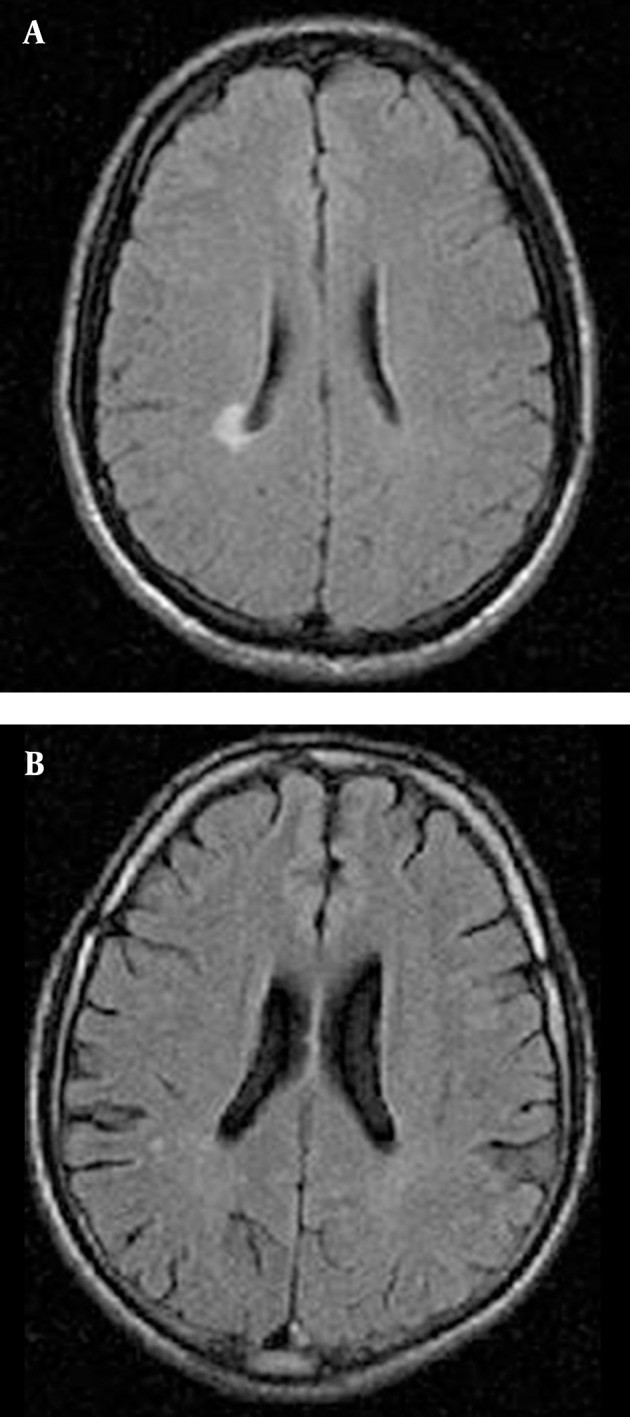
Axial FLAIR images. A) MA dependent subject, 27-year-old man with right paraventricular WM shows a large confluent area (GIII). B) Methadone dependent subject, 22-year-old woman, punctuate foci (GI) in the right parietal subcortex.
